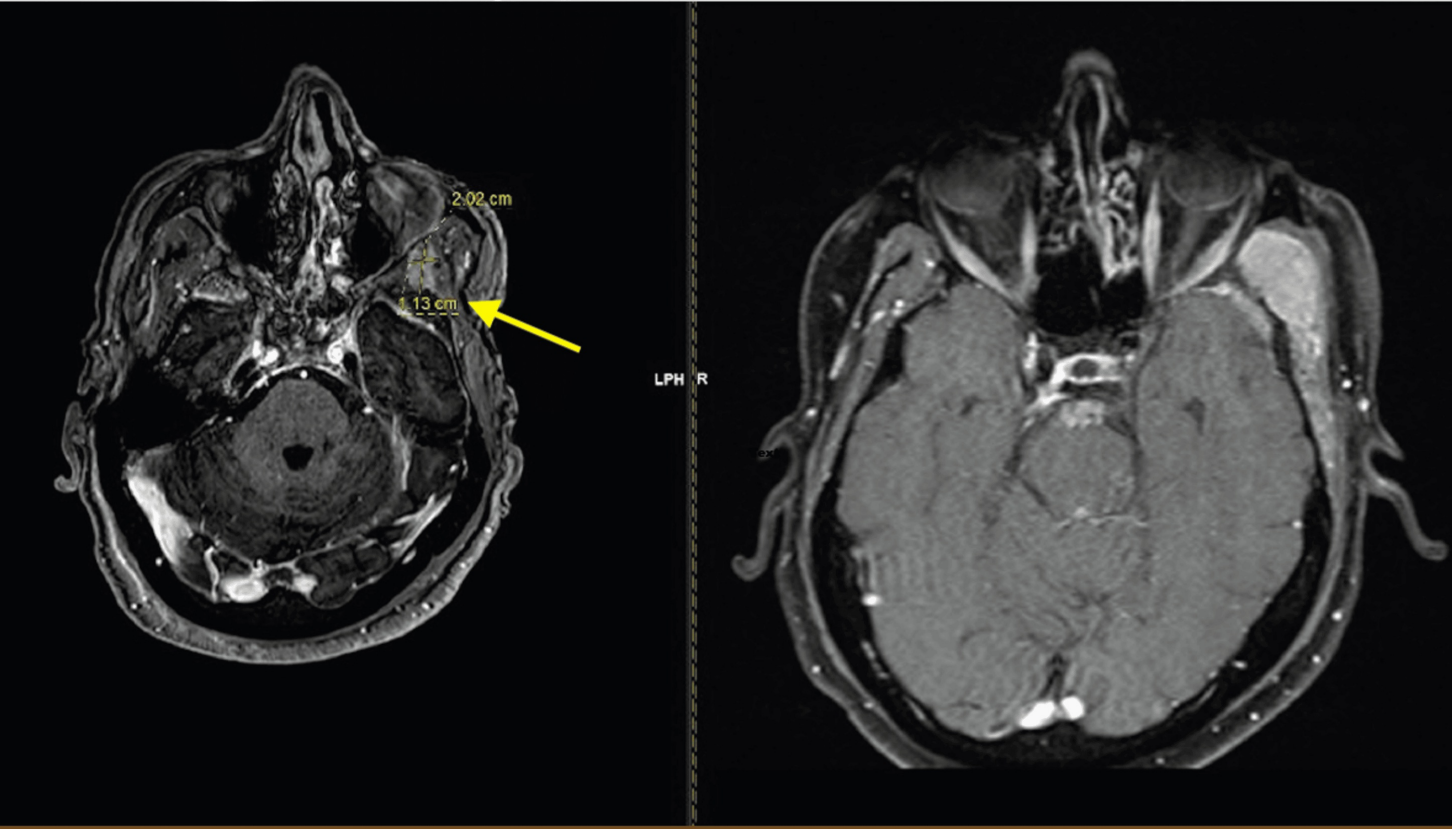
Axial MRI imaging at eight months, growth increased to 5 × 4 × 7.2 cm On the left (labelled in yellow and indicated by the yellow arrow) is the documented lesion described in the manuscript. With fat suppression (on the right), this can be better delineated and differentiated from surrounding tissue.
